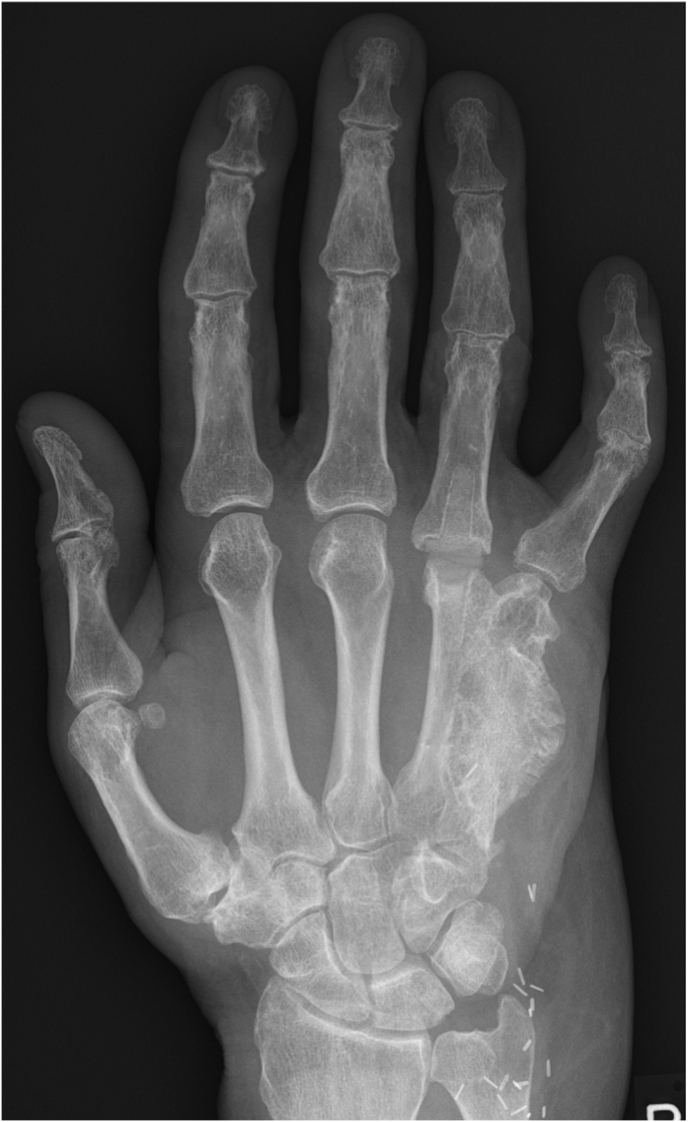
Follow-up radiograph two years after reconstruction of the 5th metacarpal and distal part of the 4th metacarpal with an osteocutaneous SCIP flap. One year after the initial trauma an MCP 4 arthroplasty was performed, placing a KeriFlex (KeriMedical, CH) implant into the completely consolidated iliac bone stock.
